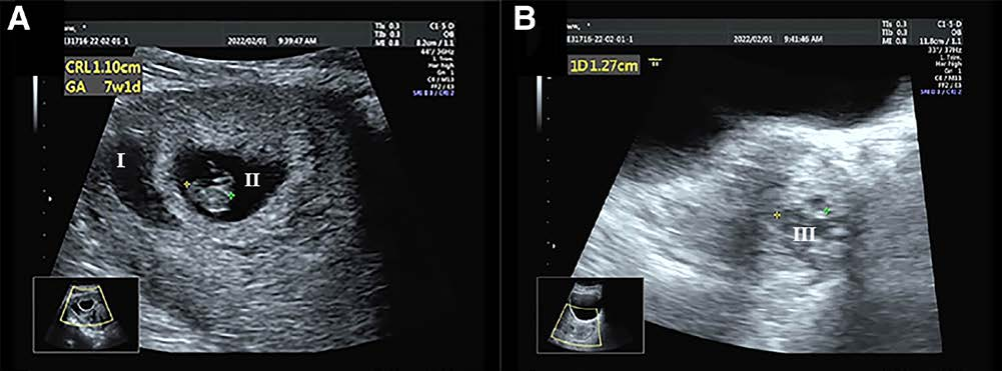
Transabdominal ultrasonography on postoperative day 1. (A) The intrauterine pregnancy sac, fetal bud, and fetal heartbeat can be seen (I); the length of the fetal bud is approximately 1.1 cm. The dark liquid area around the gestational sac is approximately 3.2 × 3.1 × 2.2 cm, with poor internal sound transmission and a ground-glass appearance (II). (B) A small amount of dark liquid area of 1.4 × 1.3 × 1.0 cm can be seen in the upper part of the cervix (III), and a separated echo can be seen.
